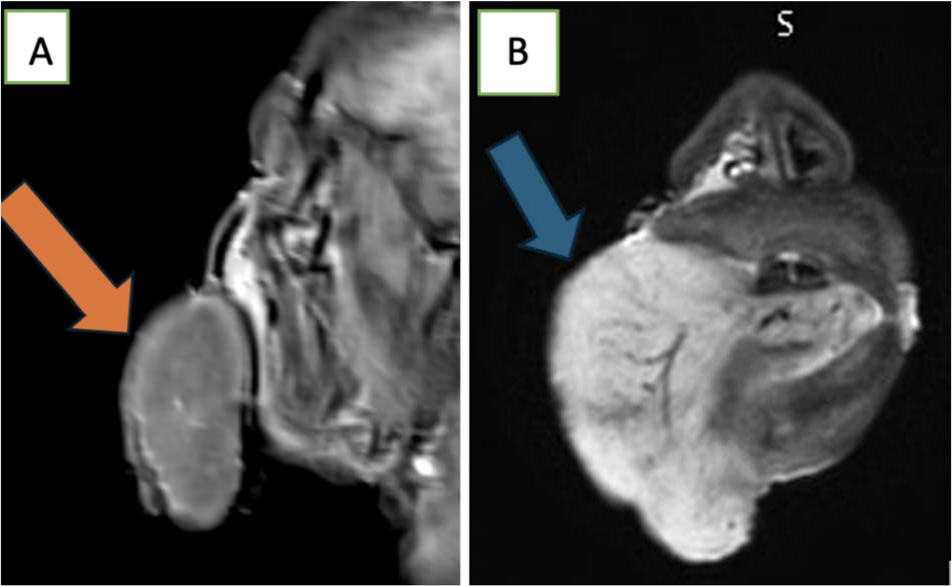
MRI Tongue shows significant macroglossia as seen in the (A) and (B) above with significant vascular engorgement these MRI features along with its subacute evolution are suggestive of being angioneurotic.
